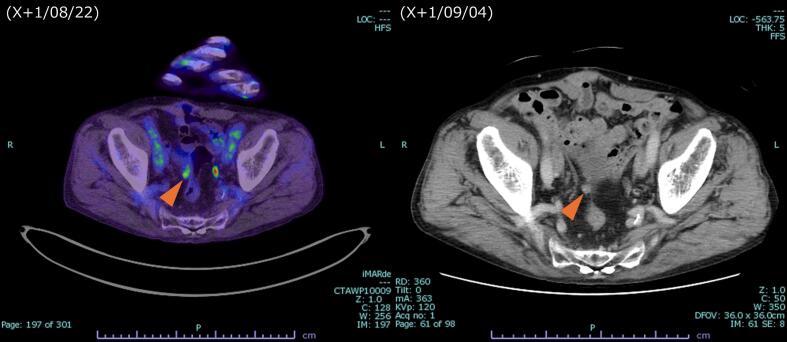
Recurrent peritoneal nodule. PET-CT demonstrated FDG accumulation (SUVmax: 3.8) at the anterior rectal wall, indicating recurrent peritoneal metastasis.
